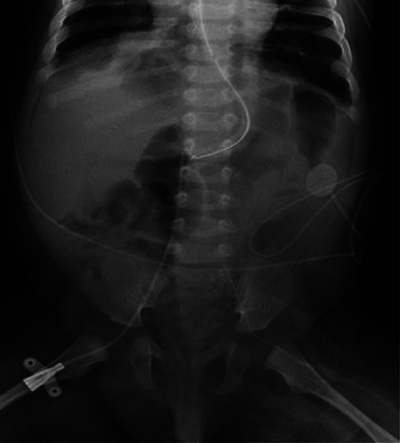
Abdominal radiograph. Hyperinflation and lateral abdominal subcutaneous emphysema.
